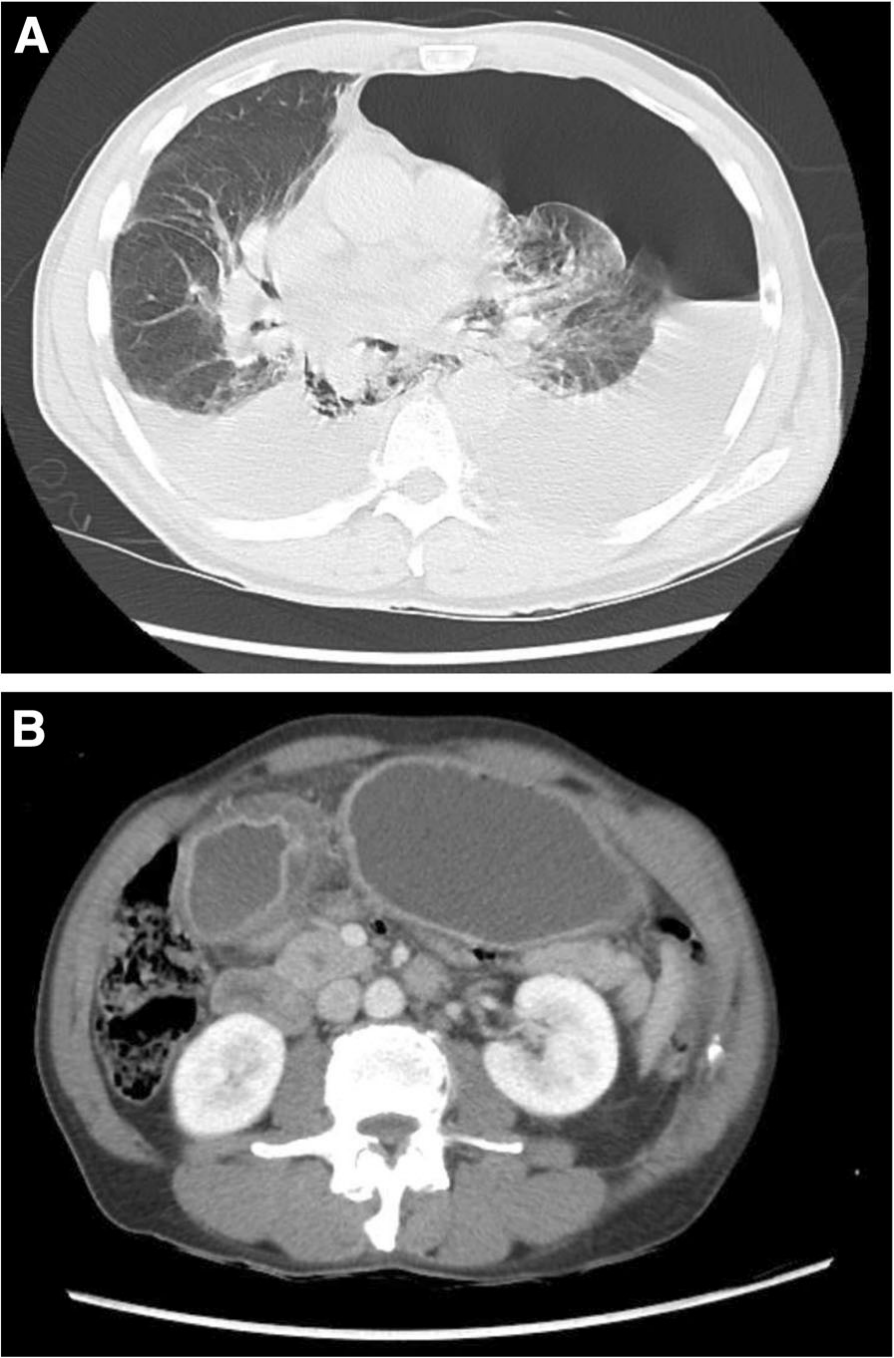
CT of the chest, abdomen, and pelvis with intravenous contrast. a Pneumomediastinum, left hydropneumothorax with rightward mediastinal shift, and right pleural effusion. b The distended, fluid-filled stomach with thickening of the pylorus consistent with gastric outlet obstruction
